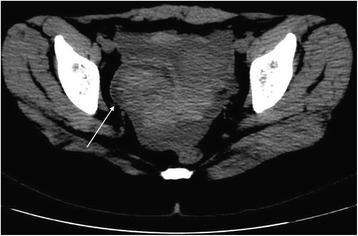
CT findings of ROCLC. CT image of a patient who presented with persistent abdominal pain for a period of 2 hours with no clear cause; HCG test was negative. Culdocentesis revealed signs of active bleeding. Single arrow indicates the ruptured corpus luteal cyst filled with high-density blood
